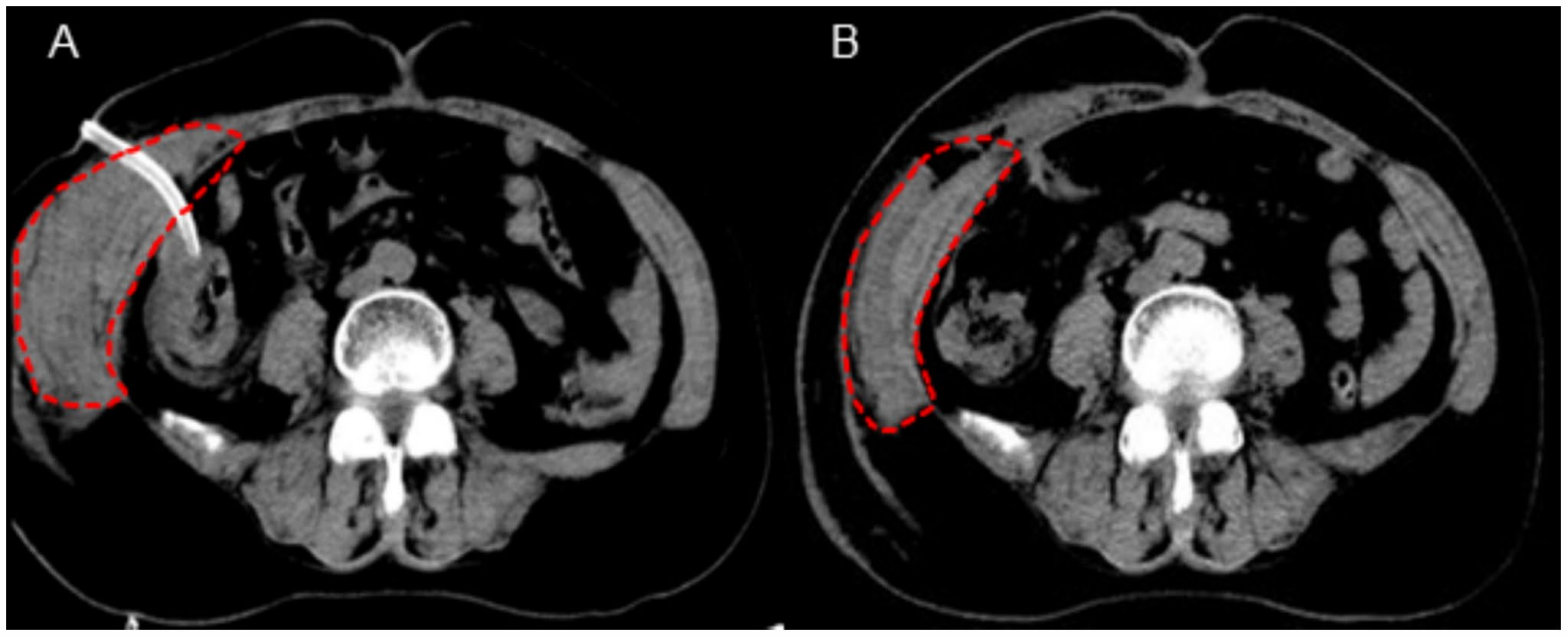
CT imaging findings of the abdominal wall hematoma (A: postoperative day 2 evaluation, B: postoperative day 5 evaluation).
